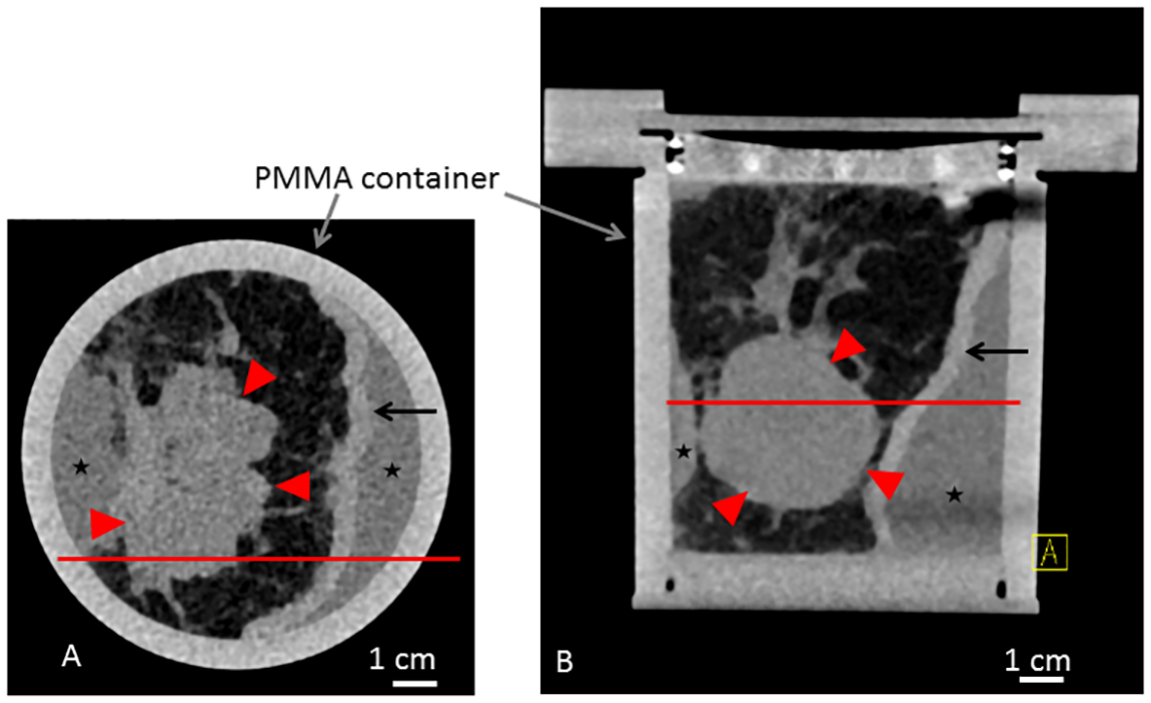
Clinical CT scan of sample A. Clinical CT scan of sample A in the sample holder with a standard clinical whole body CT scanner (Somatom Definition Flash, Siemens, Germany). Axial (A) and coronal (B) view. Red line in (A) and (B) reference for the respective imaging position. Asterisk indicating formaldehyde surrounding the sample. Red arrowheads indicating tumor. Arrows indicating skin.
